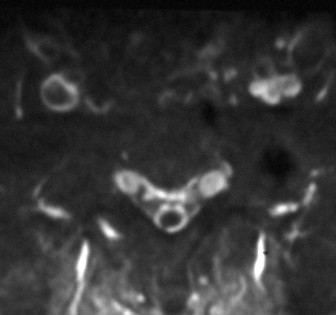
Axial section of Gadolinium-enhanced T1-weighted MRI demonstrated an annular sac from S1 level to the most caudal region of the dural sac.
